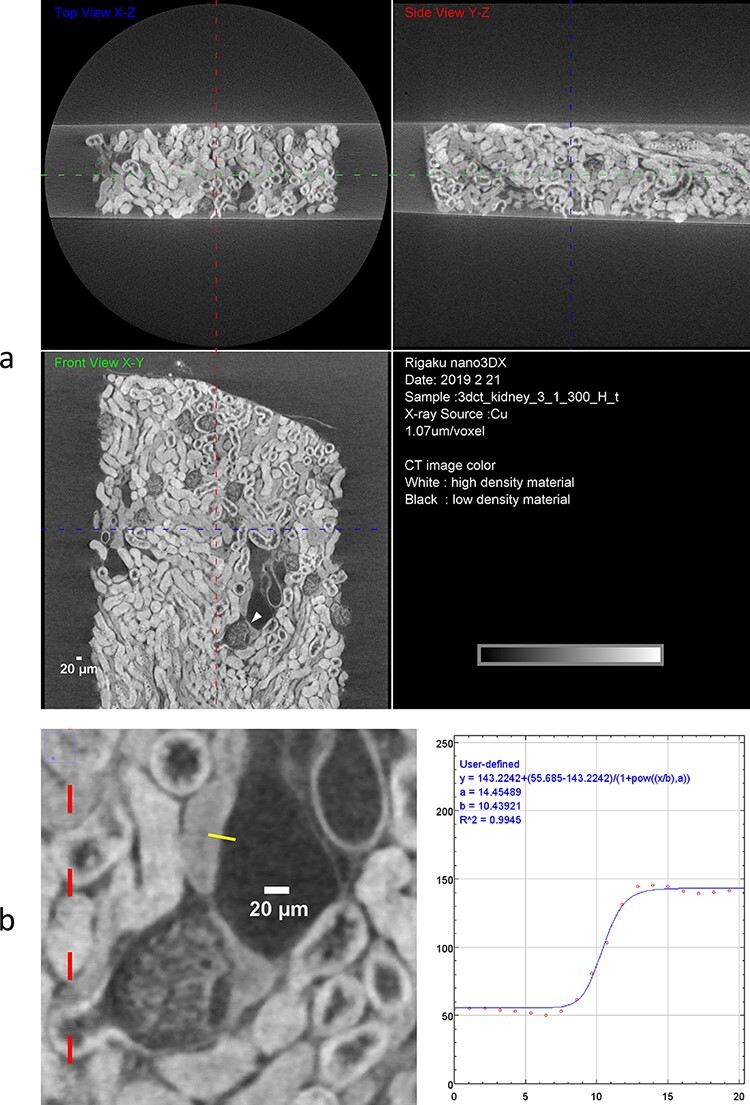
CT reconstruction. (a) Orthogonal CT slices from three different perspectives. These images are derived from the sample shown in Fig. 1a and in Table 1 (1_300_H_t). A renal corpuscle used for the nephron segmentation is indicated by an arrowhead. Conditions for the data collection are described in Materials and methods. (b) Measurement of SBE. Magnified part of (a) around the arrowhead is presented with an opacity profile along the solid line orthogonal to resin-tissue boundary (about 20 μm). Obtained SBE from the curve fitting was 1.59 μm.
